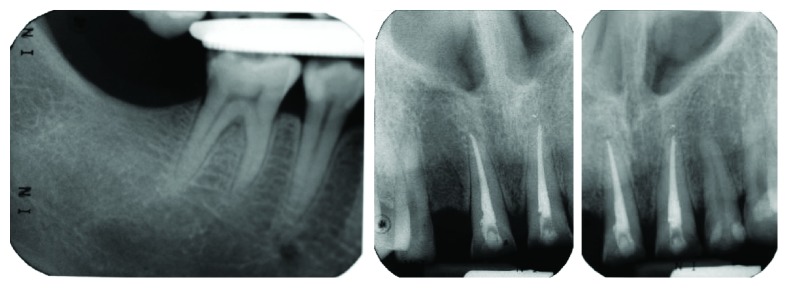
Preoperative periapical of 46 and upper anterior teeth showing no periapical radiolucency with good alveolar bone level.
